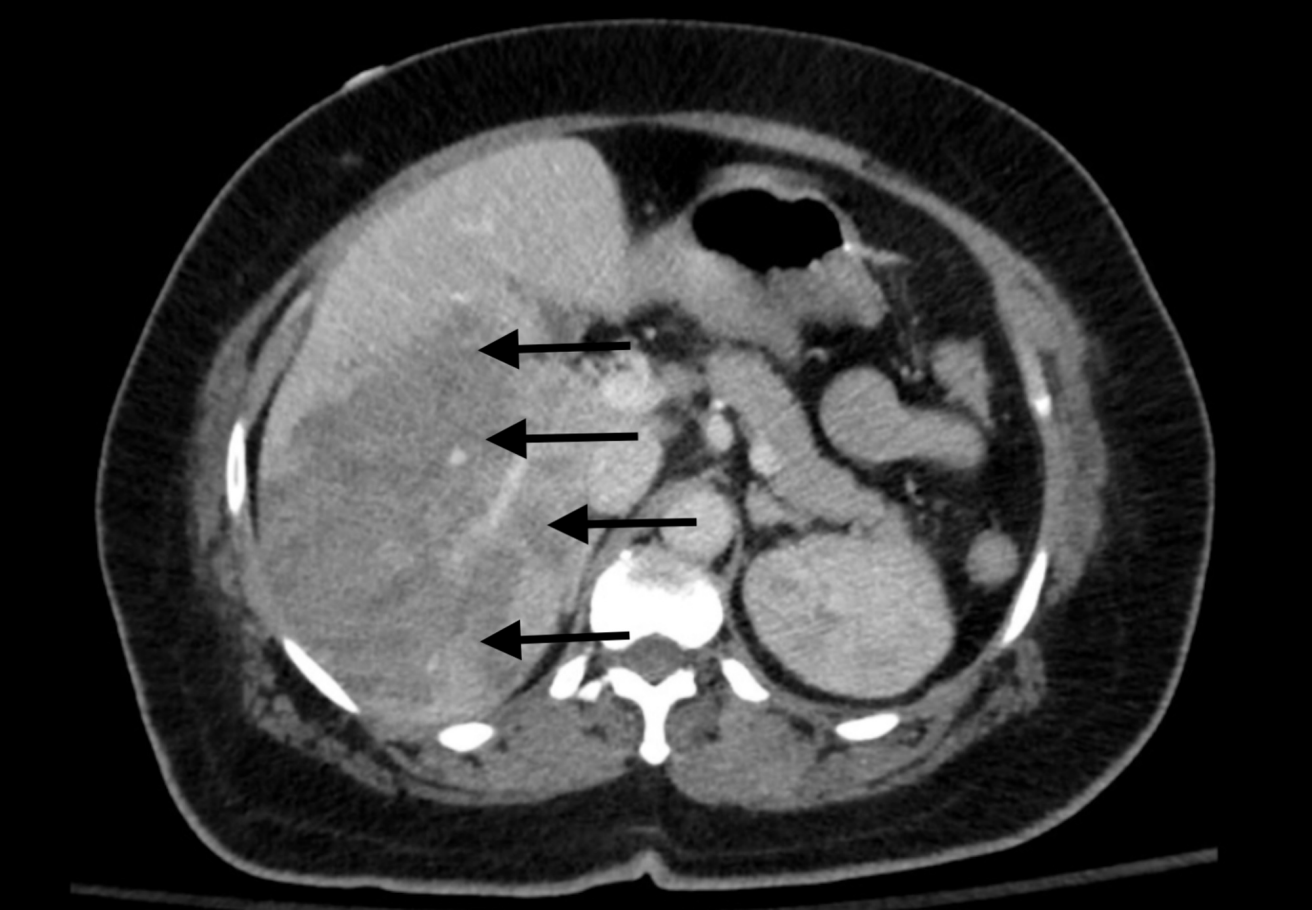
Three-phase liver CT confirming the heterogeneous area to be intra-parenchymal hemorrhage in the right side of the liver
